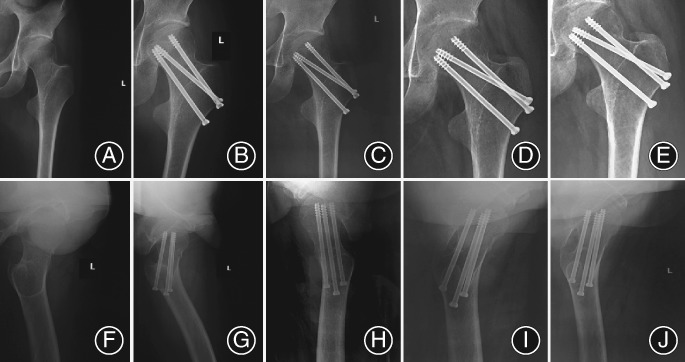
Radiograph showing fracture healing process in a 55‐year‐old woman fixed with the transverse cancellous lag screw (TCLS) technique. (A–E) The anterioposterior X‐ray review of proximal femur at 1 day, 3 months, 6 months, and 12 months after the operation. (F–J) The lateral X‐ray review of the proximal femur at 1 day, 3 months, 6 months, and 12 months after the operation.
